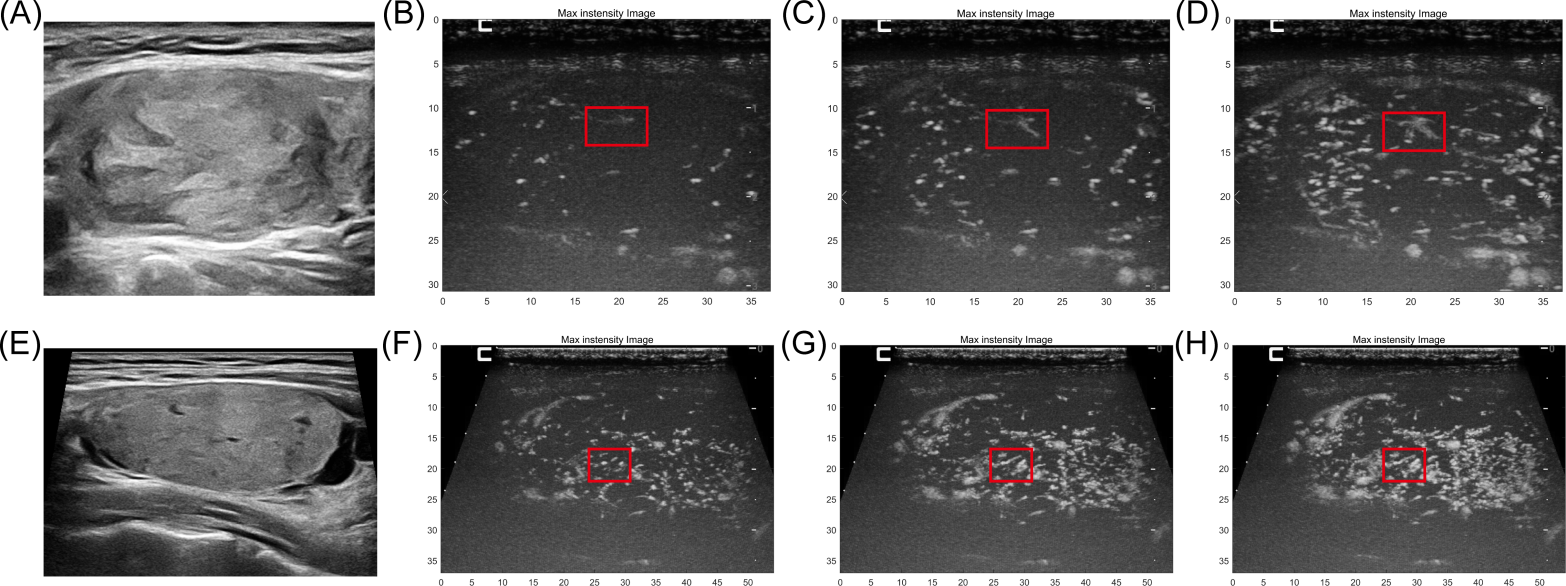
The conventional ultrasound and MIP image sequences of two cases of suspected follicular thyroid tumors with similar conventional ultrasonography characteristic. (A) B-mode ultrasound of a 44-year-old woman with FTC proven by pathology. (B–D) MIP sequences of FTC refer the microvascular with typical firework-like pattern characteristic (red box). (E) B-mode ultrasound of a 35-year-old man with FA proven by pathology. (F–H) MIP sequences of FA, microvasculature morphology shows typical parallel-, short rod-like pattern.
